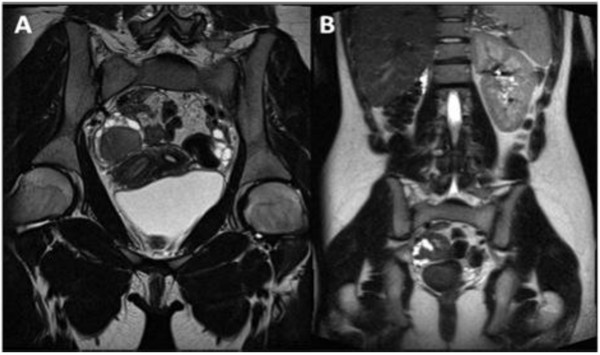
A) Oblique coronal TSE T2-weighted MR image shows a complete view of the anatomical defects. B) Coronal T2 Trufi MR image showing the omolateral renal aplasia with respect to the pelvic abnormalities and a solitary left kidney with a mild hypertrophy.
